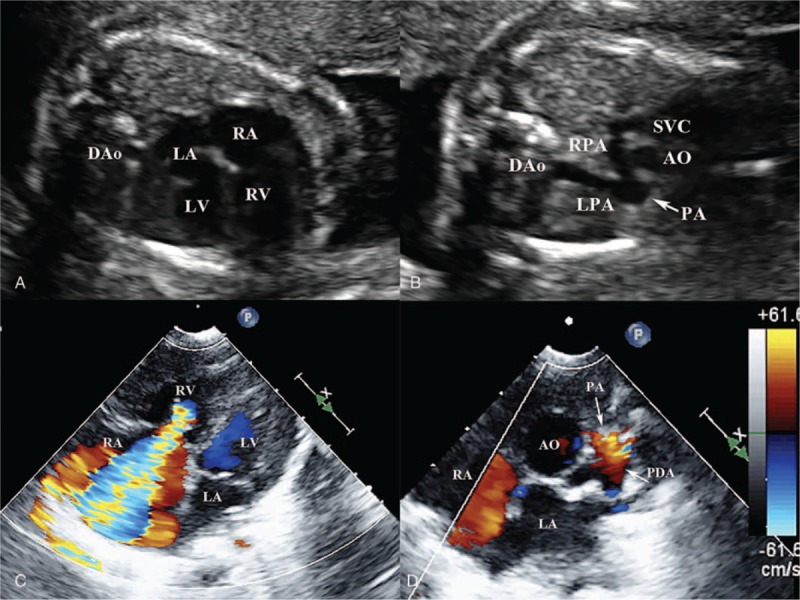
The prenatal and postnatal echocardiography of type I pulmonary atresia with intact ventricular septum. (A) The prenatal echocardiography at 23 weeks’ gestation, four-chamber view shows right ventricle is well developed, no venticulo-coronary artery communication, intact ventricular septum. (B) The 3-vessel-trachea view shows pulmonary atresia (*narrow*). (C) The postnatal echocardiography of the same fetus, four-chamber view shows right ventricle is well developed, severe tricuspid regurgitation, no venticulo-coronary artery communication. (D) Parasternal short axis shows pulmonary atresia (*arrow*), patent ductus arteriosus. AO = aorta, DAo = descending aorta, LA = left atrium, LPA = left pulmonary artery, LV = left ventricle, PA = pulmonary atresia, PDA = patent ductus arteriosus, RA = right atrium, RPA = right pulmonary artery, RV = right ventricle, SVC = superior vena cava, VCAC = ventriculo-coronary artery communication.
